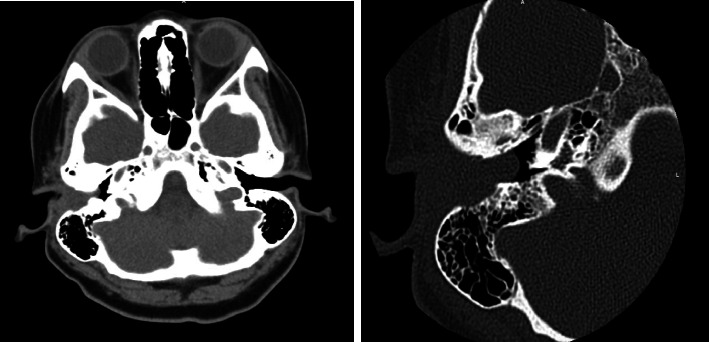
CT demonstrating obstruction of the right EAC by a soft tissue mass without erosion.
